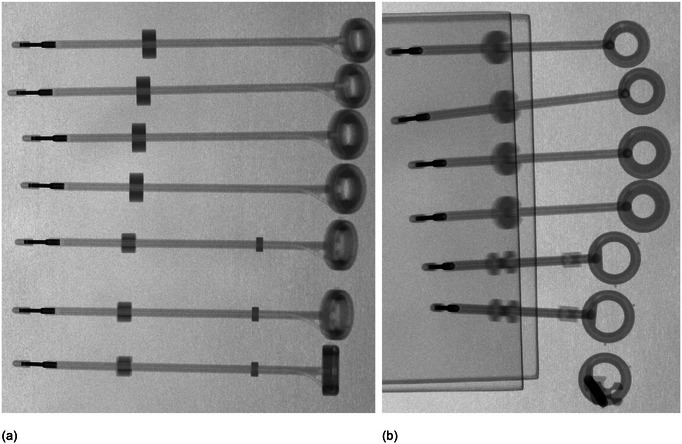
Radiographs of the ring applicators, with no marker wires in place. Applicators are arranged from top to bottom, Set A 45° ring ⌀26 mm × 32 mm, Set B 45° ring ⌀26 mm × 32 mm, Set A 45° ring ⌀30 mm × 36 mm, Set B 45° ring ⌀30 mm × 36 mm, Set A 3D 60° ring ⌀30 mm × 36 mm, Set B 3D 60° ring ⌀30 mm × 36 mm, and 3D 90° ring ⌀30 mm × 36 mm. The rings are arranged lying directly on the imager in (a), and propped such that the ring surface is flush with the imager surface in (b).
